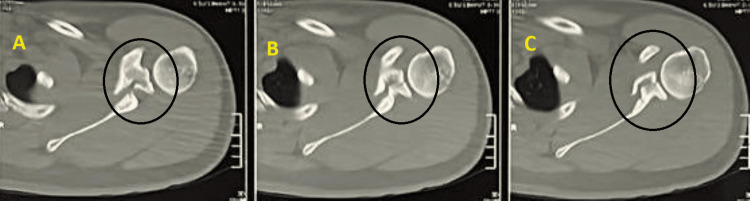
CT scan of the left shoulder joint A)-C) Serial axial cuts of the left shoulder joint The black circle shows the fracture site
